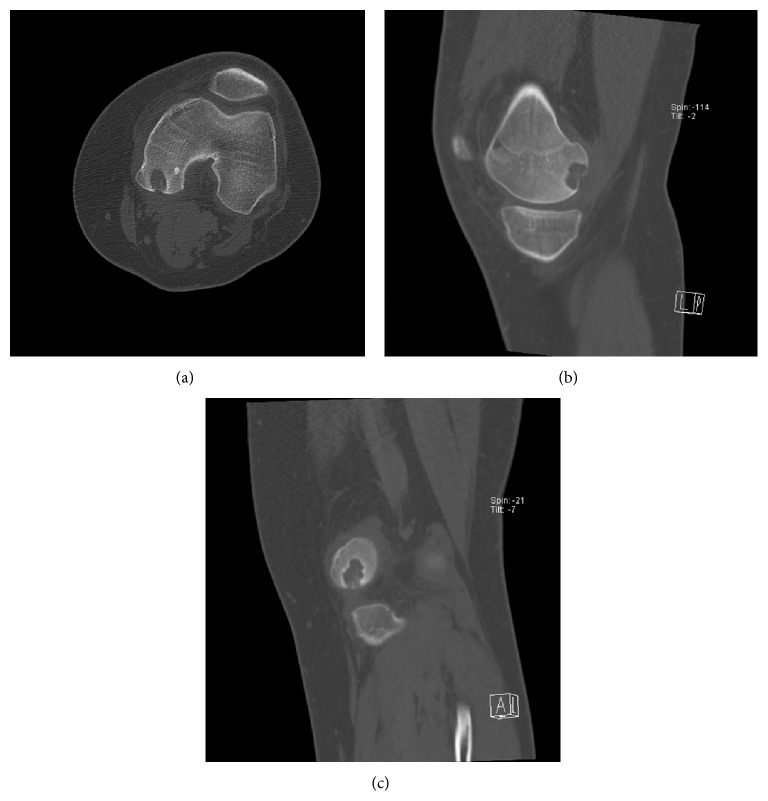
Axial (a), sagittal (b), and coronal (c) images from preoperative CT scan demonstrating a lytic lesion in the medial femoral condyle with lobulated well-defined margins and depression of the subchondral bone at the lesion border.
